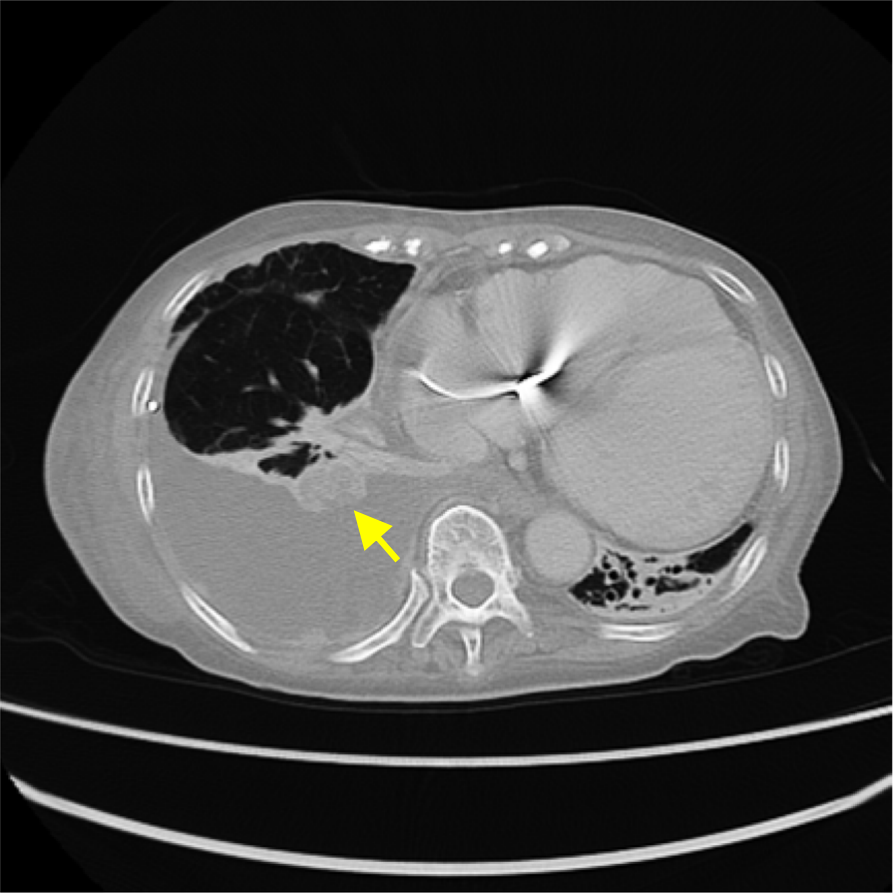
Chest CT indicated the presence of a right lung (S10) mass (arrow) and pleural dissemination
